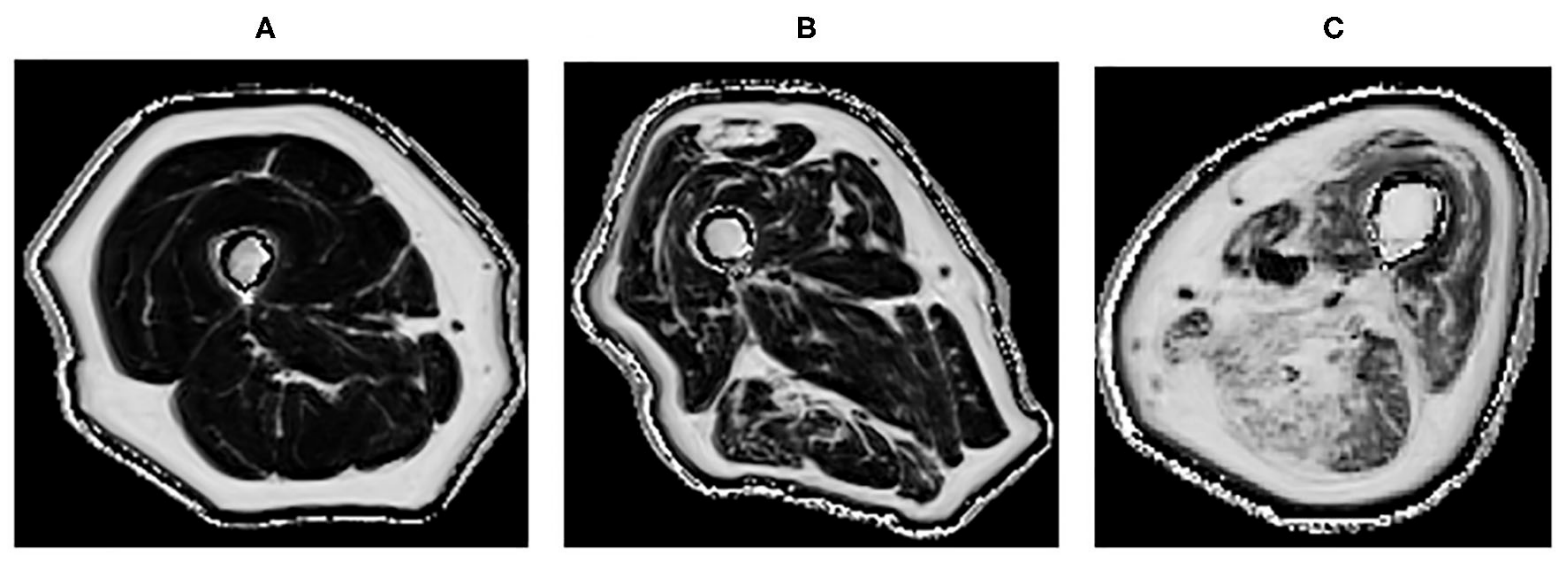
Quantitative MRI fat fraction measurement in the quadriceps and hamstrings, respectively in the thigh in (A) 45-years-old healthy female with a fat fraction of 1.9 and 2.7%, respectively, (B) 83-years-old healthy male presenting with fatty infiltration associated with healthy aging with a fat fraction of 9.6 and 13.4%, respectively, (C) 60-years-old male with active myositis presenting with fatty infiltration with a fat fraction of 19.6 and 28.5%, respectively.
